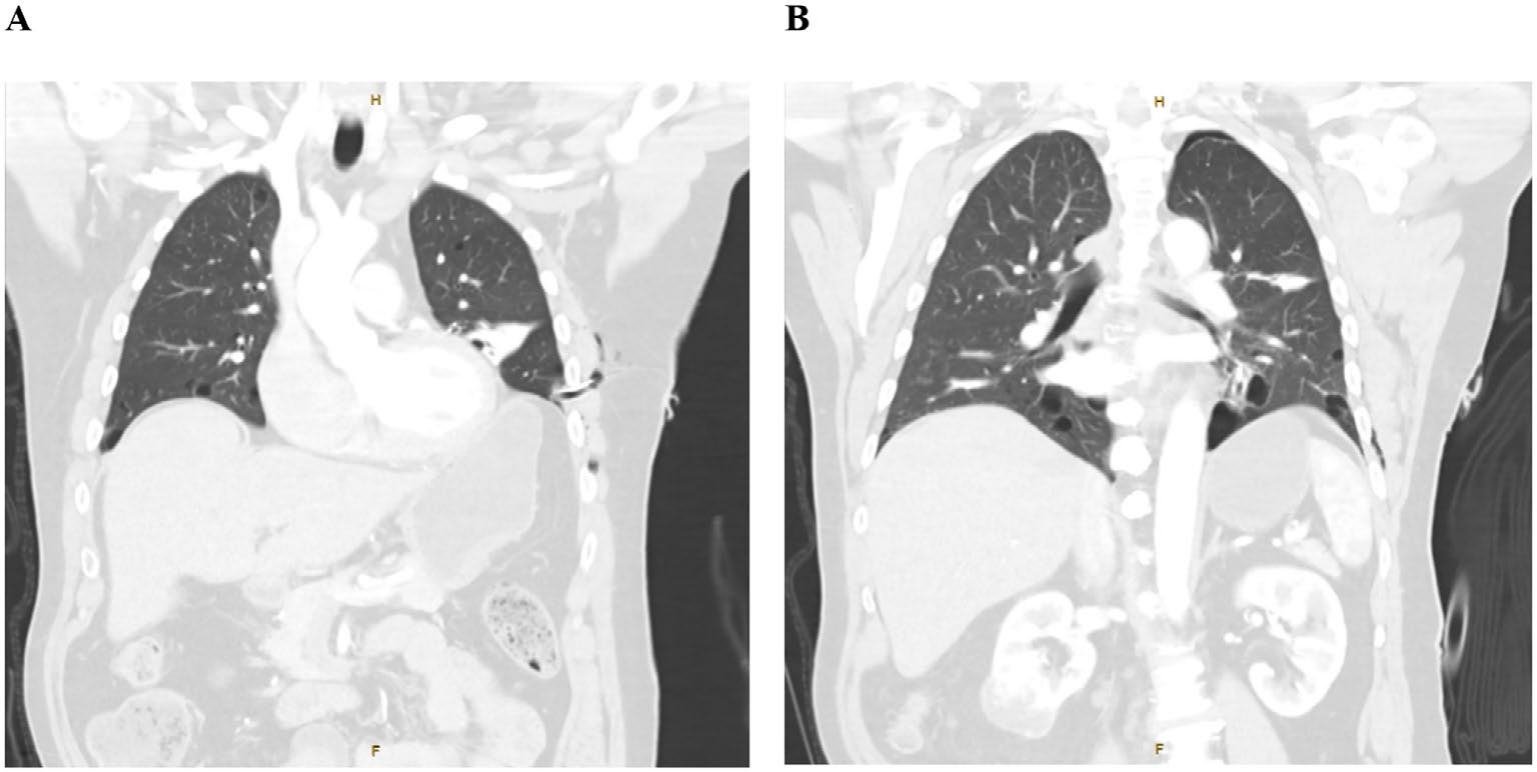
(A) Computed tomography scan of the chest in the coronal plane indicating the presence of an appropriately sited pigtail intercostal catheter entering the left pleural cavity. (B) Computed tomography scan of the chest in the coronal plane indicating the presence of a small residual apical pneumothorax following the insertion of a pigtail catheter.
